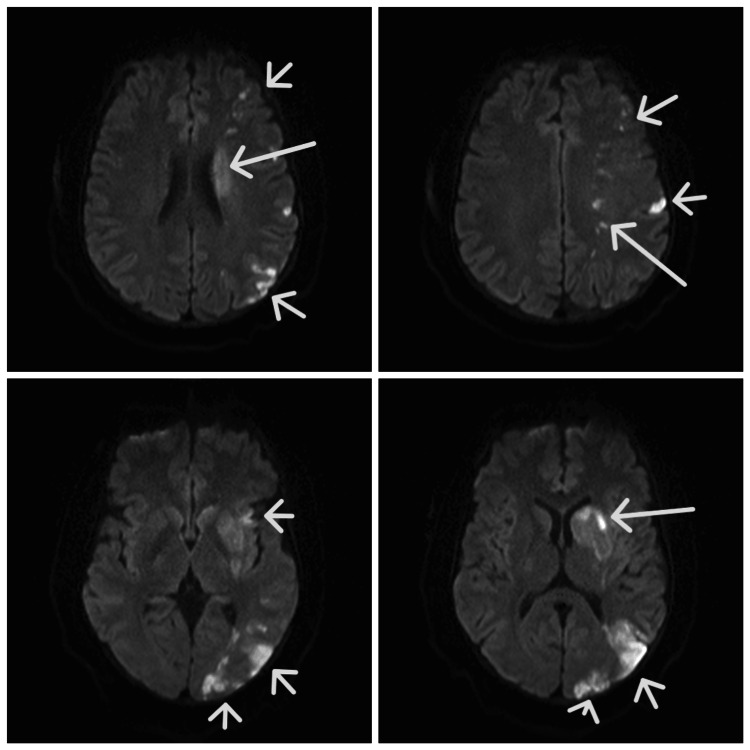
MRI brain DWI showing left MCA territory infarct (see arrows) DWI: Diffusion-weighted Image, MCA: Middle cerebral artery
